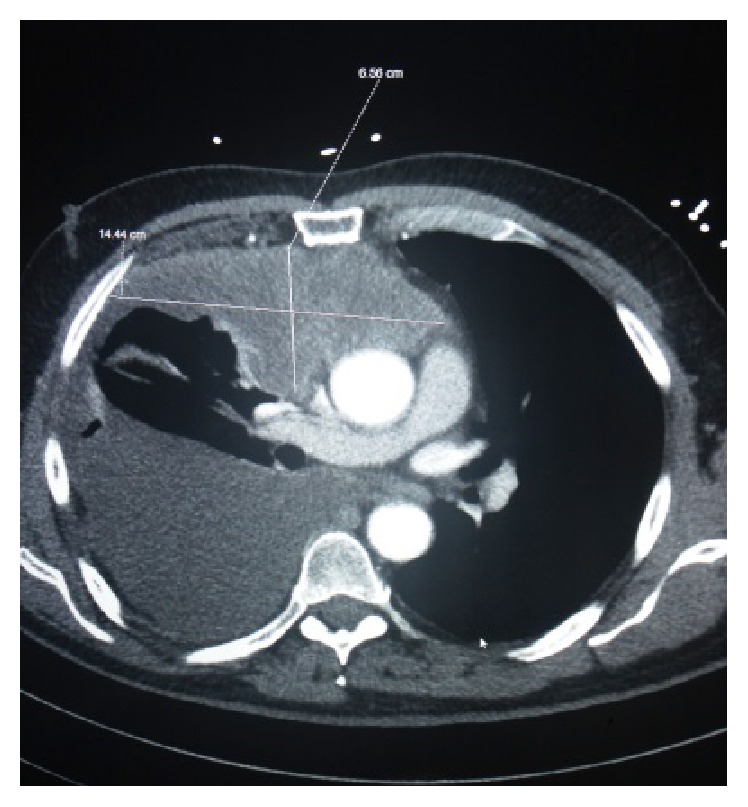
Computerized tomography (CT) scans of chest revealed a large mass/conglomerate lymphadenopathy measuring 6.6 cm × 14.5 cm × 7 cm in the anterior inferior mediastinum extending to involve the anterior right hemithorax, along the pleural surface, associated with a very large right-sided pleural effusion, causing partial collapse of the right inferior and middle lobes.
